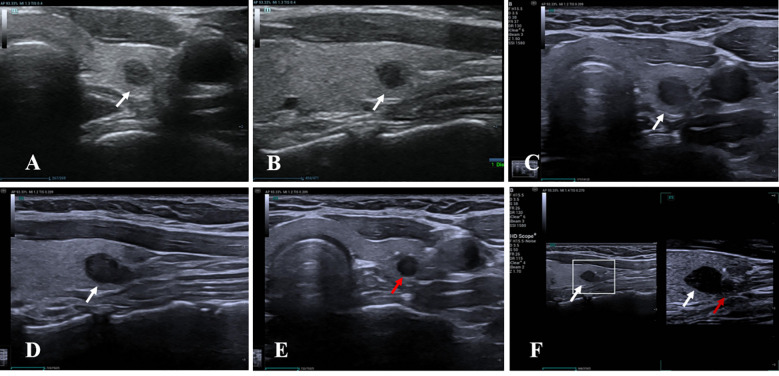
Development of a new suspicious lesion during active surveillance without significant primary nodule growth. A 37-year-old woman was initially diagnosed with a ACR TI-RADS 5 nodule. Baseline transverse (A) and longitudinal (B) ultrasound images show a suspicious nodule measuring 4.0 × 3.9 × 3.3 mm. At the 18-month follow-up, transverse (C) and longitudinal (D) images show mild growth of the original nodule to 6.3 × 5.1 × 5.4 mm; however, the increase did not exceed the 3 mm threshold in any single axis. Meanwhile, a new suspicious lesion measuring 2.3 × 3.2 × 3.7 mm appeared inferior to the original nodule. Transverse view (E) demonstrates the new lesion, while high-resolution longitudinal imaging (F) shows the spatial relationship between the original and new nodules. White arrows indicate the original nodule; red arrows indicate the newly developed lesion.
